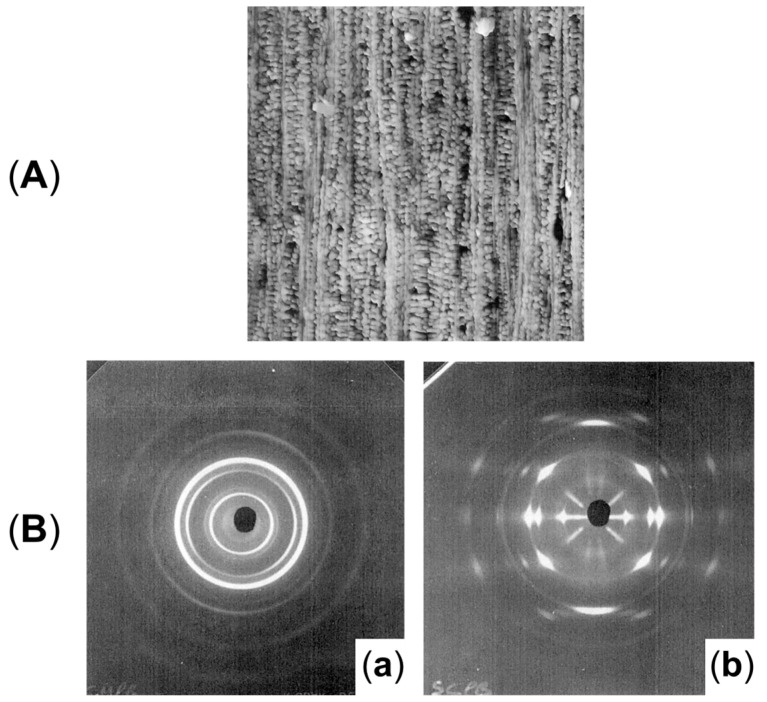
(A) AFM image of the SCORIM sample (reported area of 5 × 5 μm and 200 nm as z-scale). (B) X-ray patterns of (a) CIM and (b) SCORIM PB. [8]. Reprinted with permission from John Wiley and Sons, 2003.
